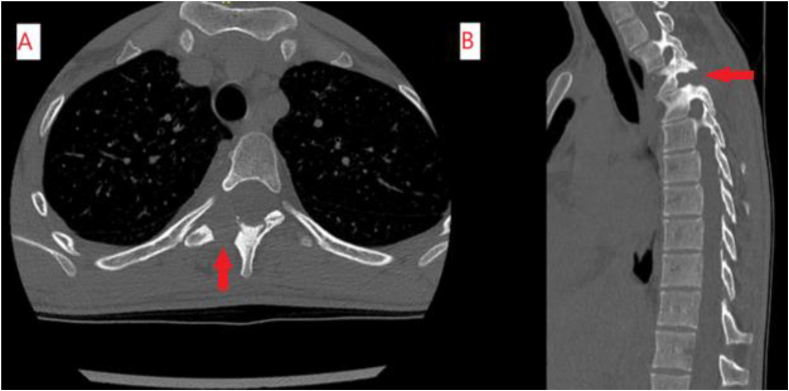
Follow-up CT scan at 1 month postoperatively demonstrating complete resection of the lesion with no residual tumor (red arrows) at the right T4-5 facet joint region. (A: axial cut; B: sagittal cut).
